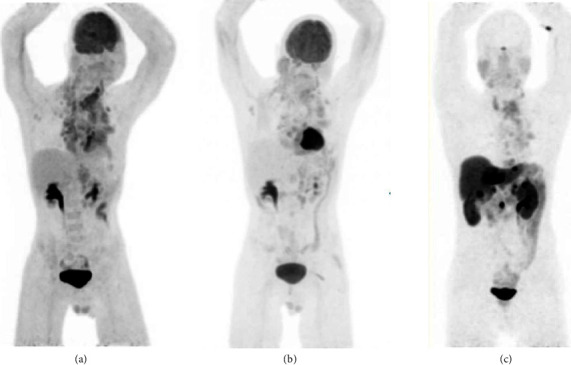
Fluorodeoxyglucose (FDG). (a) August 2020 and (b) December 2020 versus Gallium-68 DOTATATE (GATATE), and (c) positron emission tomography (PET). FDG avidity of the mediastinal mass and left perinephric mass implies a more aggressive and less differentiated disease. DOTATATE avidity of liver and pancreatic lesions implies well-differentiated neuroendocrine tumours.
